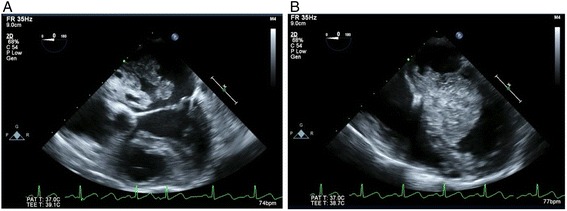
Four-chamber transesophageal echocardiogram of the large left atrial myxoma during systole (a) and diastole (b)
